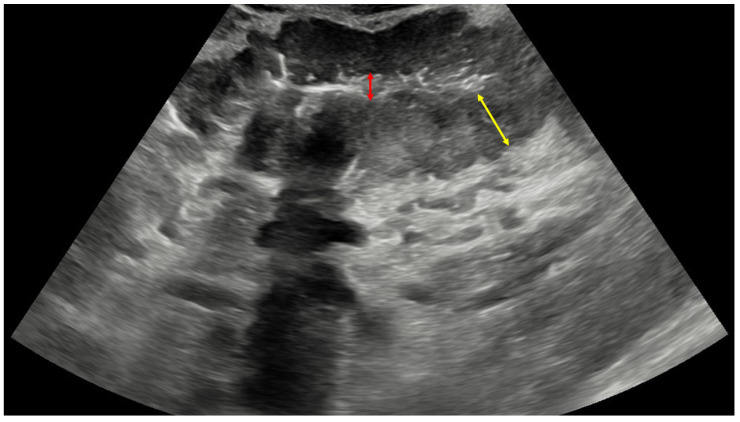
Ultrasound image of pseudokidney sign in this patient with the centrally located hyperechoic gastric lumen (red arrow) surrounded by hypoechoic and thickened gastric wall (yellow arrow), indicative of gastric cancer. Additional findings that may be present in such cases are hypervascularity of the mass, enlarged lymph nodes, and hyperechoic surrounding mesenteric fat.
